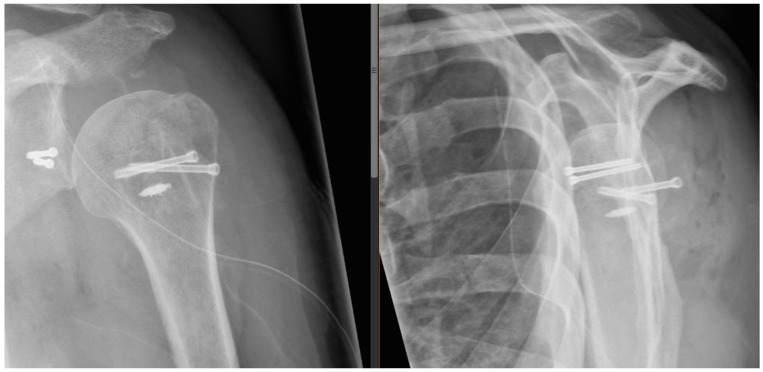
Postoperative radiographs. Anteroposterior and lateral “Y-view” radiographs showing the postoperative situation.
